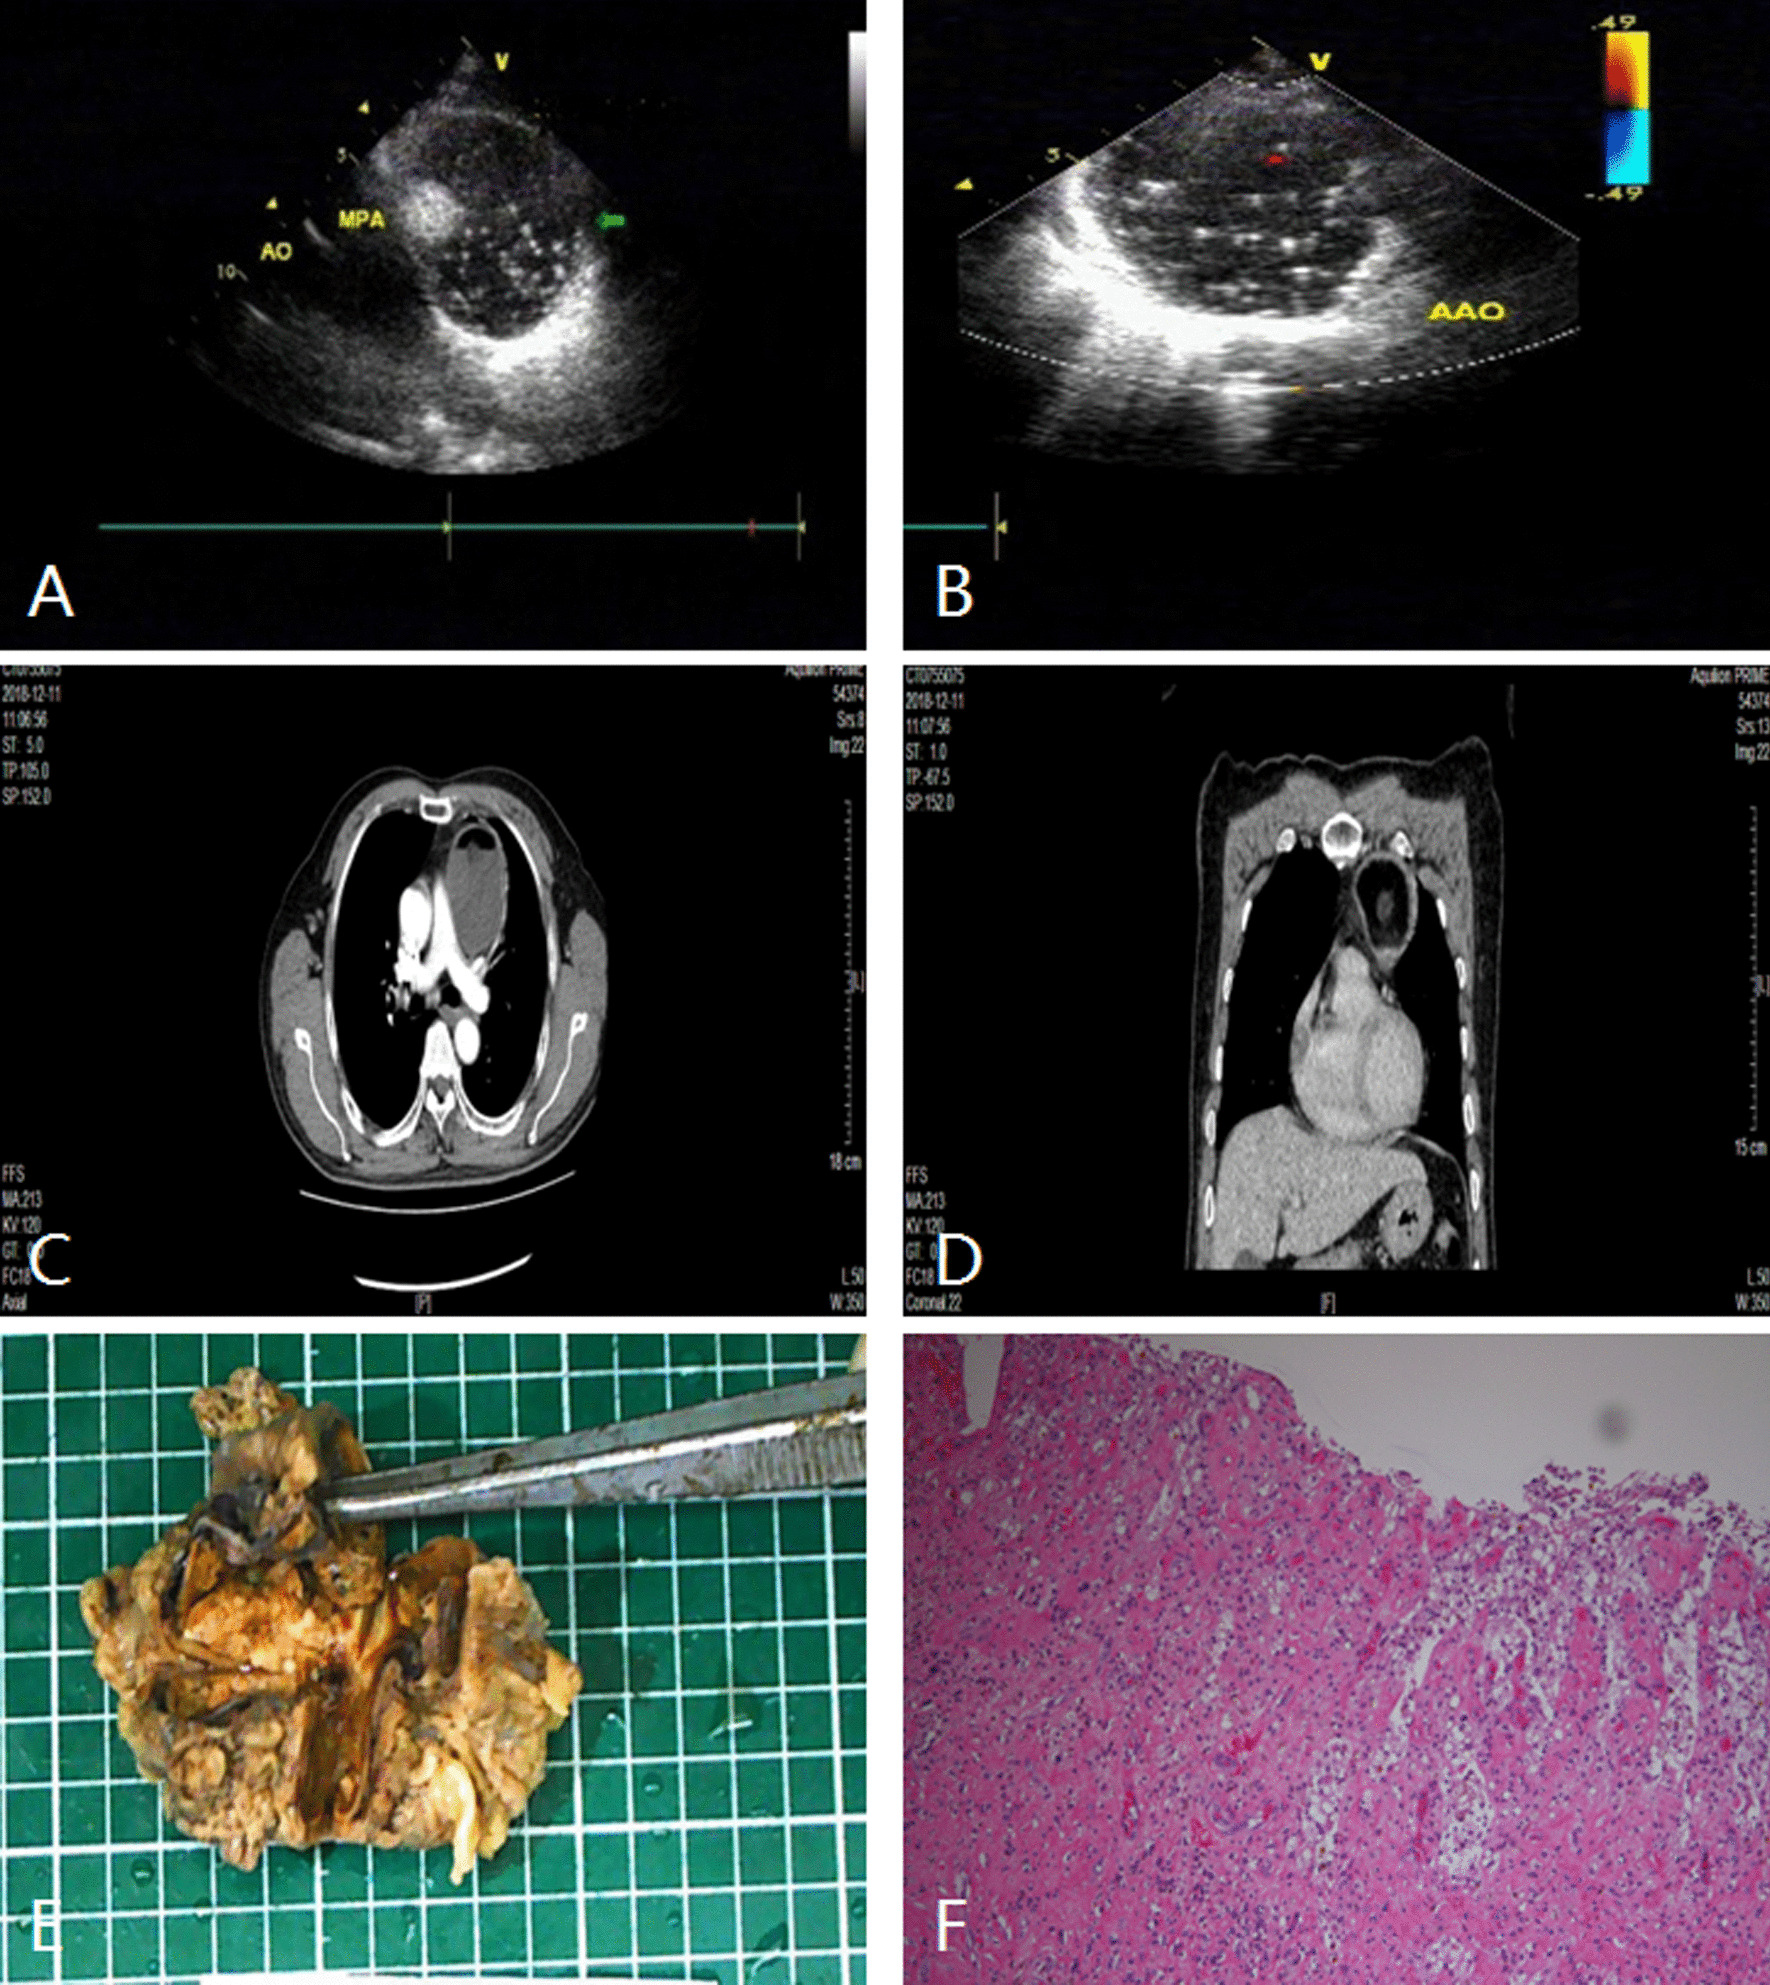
Anterior mediastinal mass (teratoma) in a 59-year-old man. A and B TTE showed a well-circumscribed round cystic-solid mass in front of the aorta and pulmonary artery. C and D Contrast-enhanced CT showed a round like abnormal density shadow with smooth boundary, with liquid and fat density shadow in it, presenting as fat liquid plane, with slightly thick wall and annular calcification and no obvious enhancement. E and F The pathologic diagnosis of this mass was mature cystic teratoma. The wall of the capsule is thick and the structure of bean curd residue can be seen in it. TTE indicates transthoracic echocardiography; CT indicates computer tomograph; AO indicates aorta; AAO indicates ascending aorta; PA, pulmonary artery
